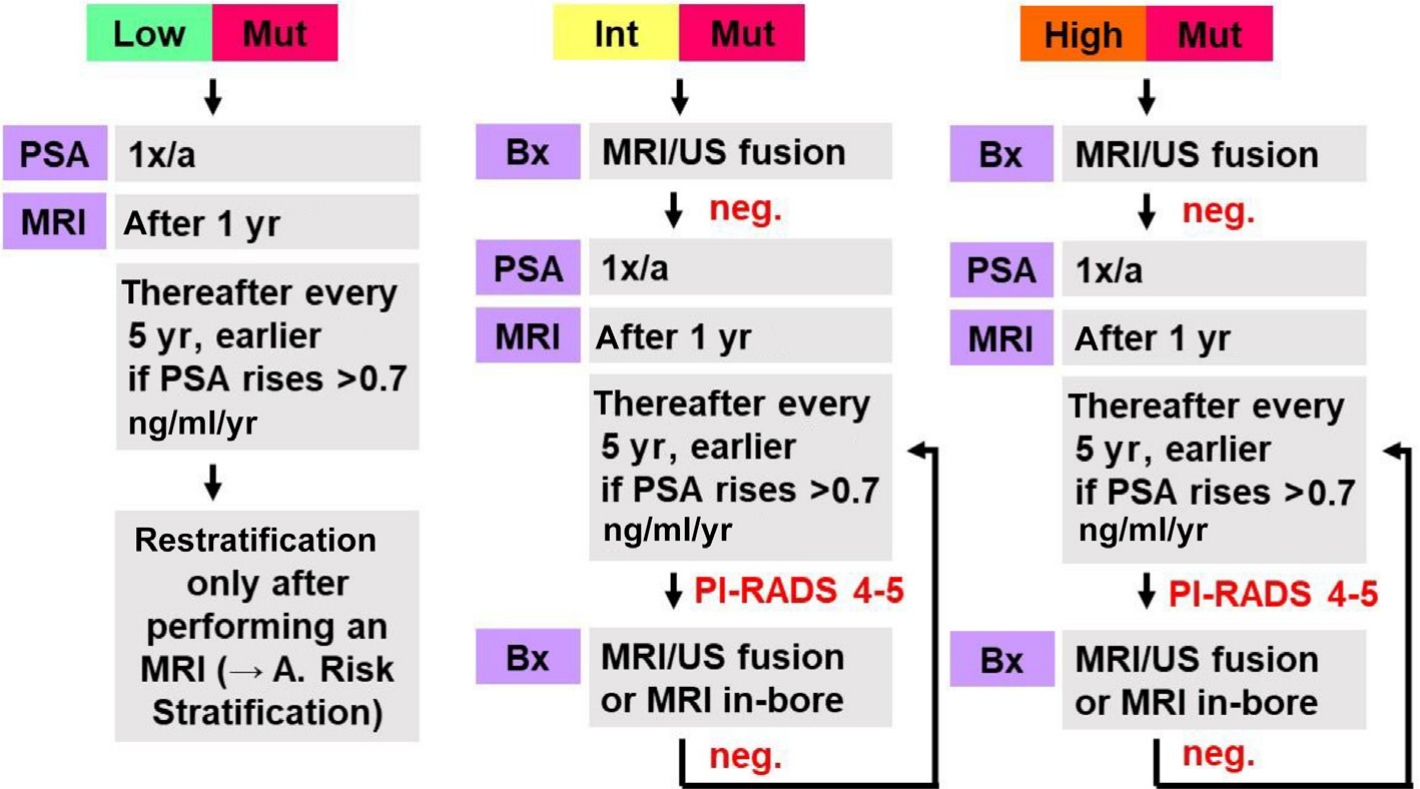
(A) Risk stratification in groups 1 and 2. (B) Follow-up management in groups 1 and 2. Bx = biopsy; ERSPC-RC4 = European Randomized Study of Screening for Prostate Cancer risk calculator 4; High = high risk; Int = intermediate risk; Low = low risk; MRI = magnetic resonance imaging; Mut = mutational burden; neg. = negative; PI-RADS = Prostate Imaging Reporting and Data System (version 2.1); PSA = prostate-specific antigen; US = ultrasound; 1x/a = once per year.
